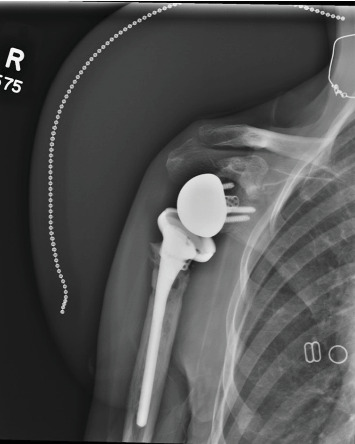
A 6-month postoperative radiograph of the right shoulder showing intact rTSA components with evidence of new osseous irregularity along the humeral stem.
